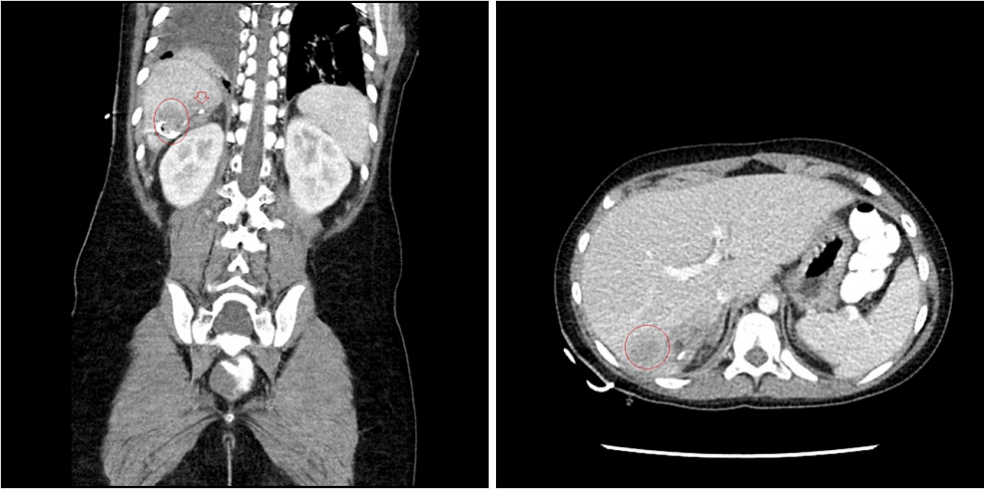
Computed tomography image showing a hepatic abscess taken on the fifth day post-drainage Along the inferior border of the abscess cavity, laying along previously noted calcifications, coiling excess drain tube can be seen as a slightly spiraled area of radiolucency within the red circle shown in the coronal view. Compared to prior images, the abscess volume is greatly reduced. The red arrow points to the previously observed area of radiolucency, thought to be a clip, which had remained in place superomedial to the main lesion throughout admission at the time of imaging.
